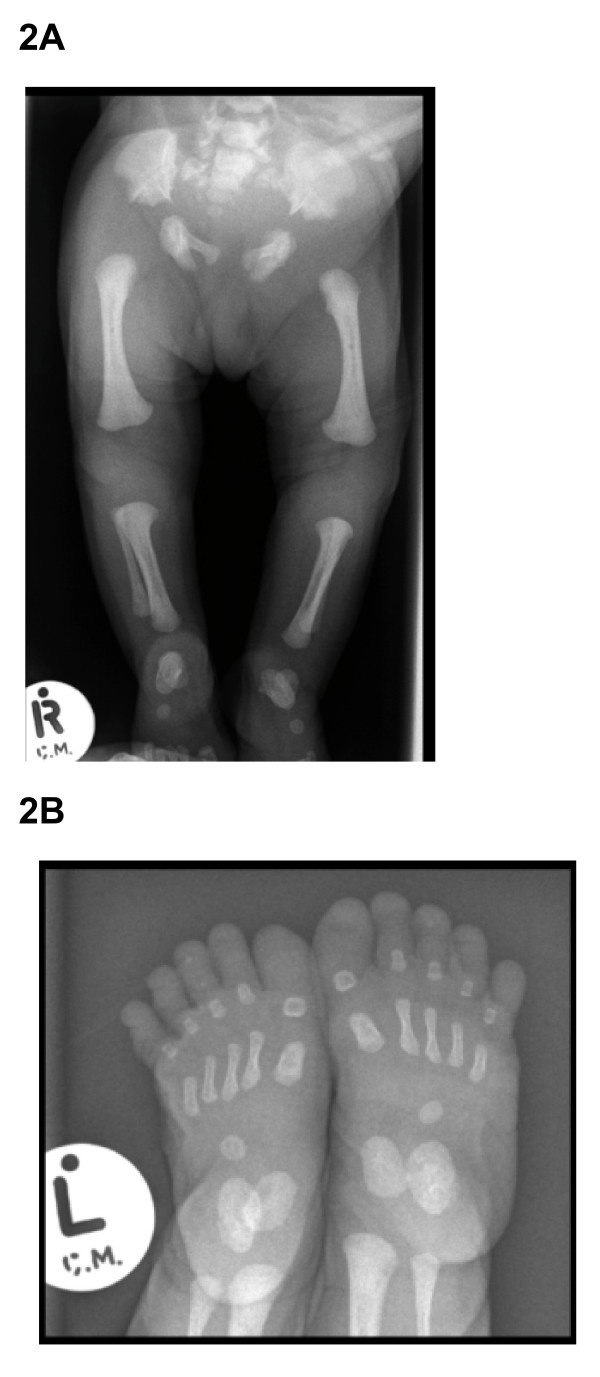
A X-ray of the lower limbs in case 3, note small iliac bones with triradiant acetabulum, short and thick long bones. 1B) X-ray of the feet in case 3 showing hypoplasia of the terminal phalanges.
